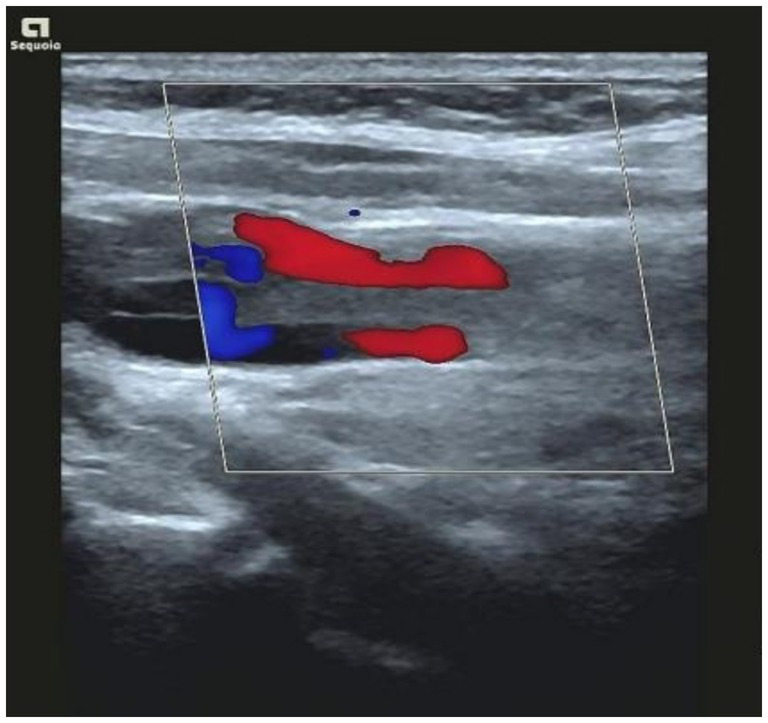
Bedside color Doppler ultrasonography suggested thrombophlebitis of the internal jugular vein.
